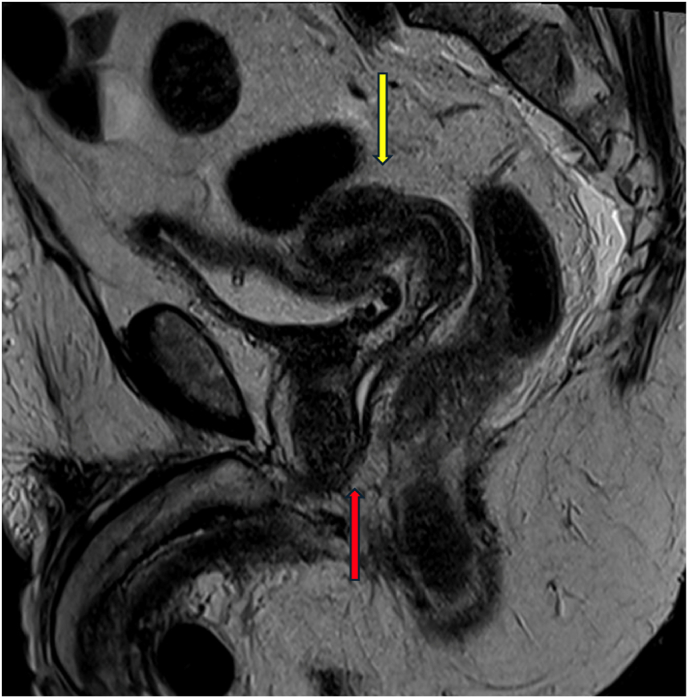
Pelvic magnetic resonance imaging showing uterus (yellow arrow) and urogenital sinus (red arrow).
